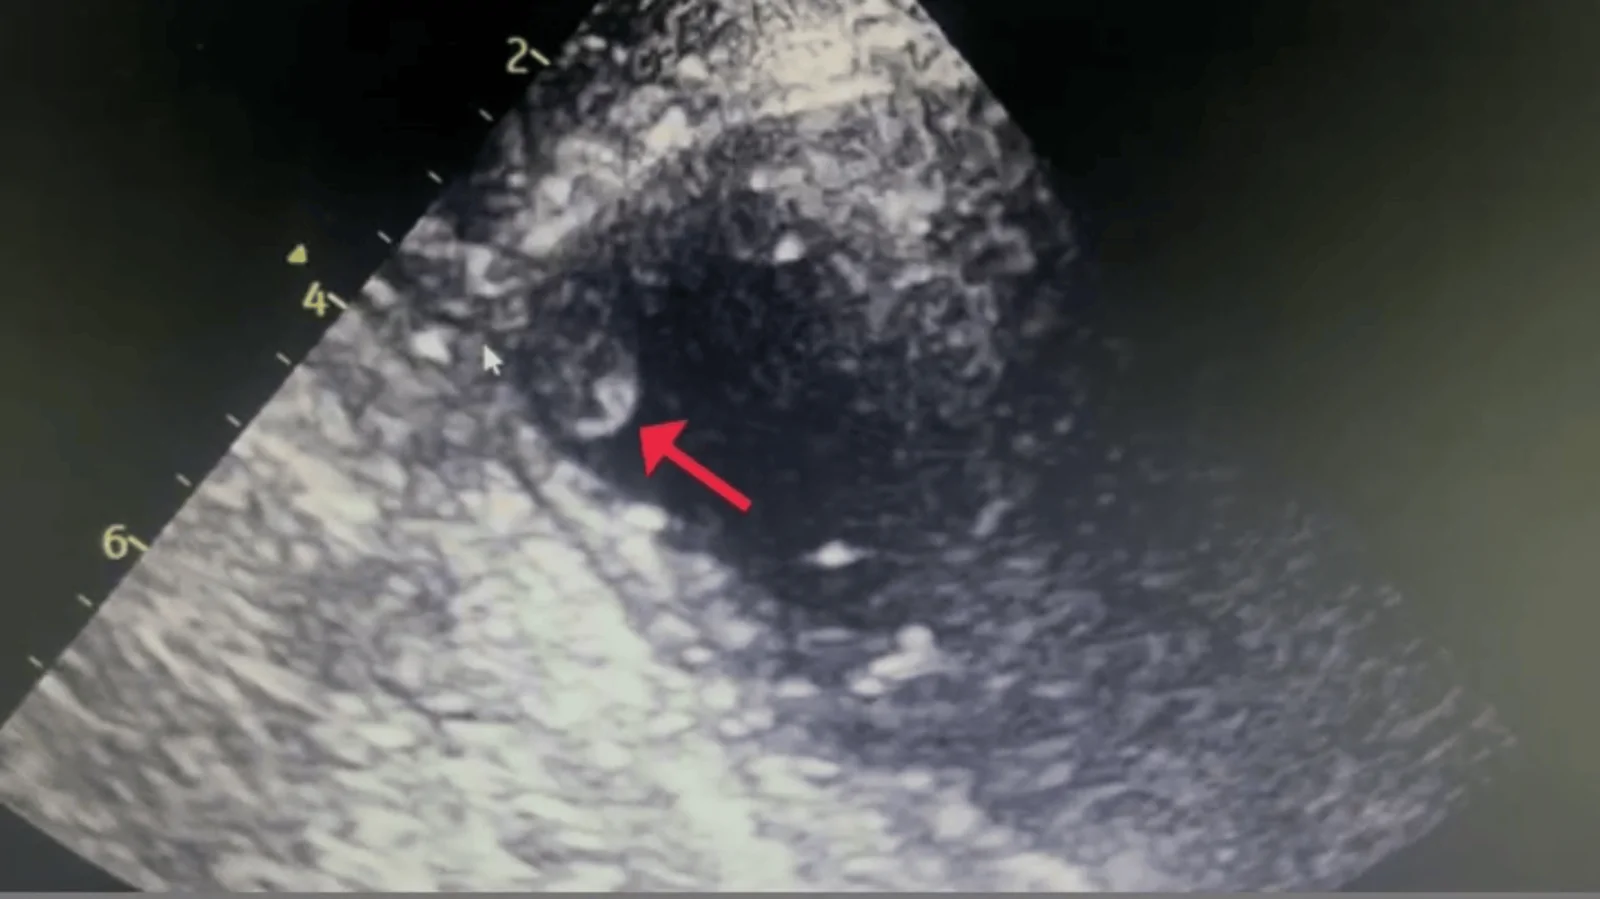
Transthoracic echocardiographic image demonstrating a left ventricular apical thrombus (red arrow) at the left ventricular apex in a patient with ischemic cardiomyopathy following anterior myocardial infarction.
